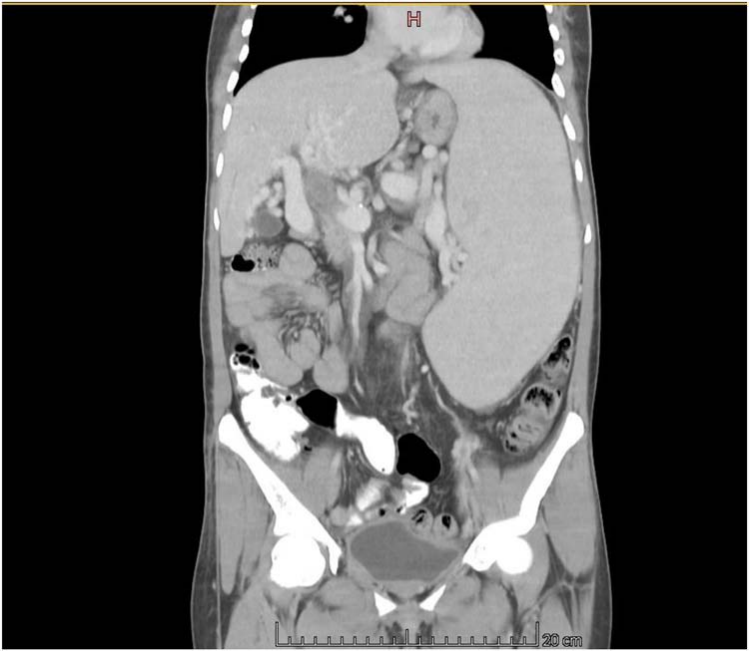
CT scan coronal view showing gross hepatosplenomegaly with large collaterals suggestive of portal venous hypertension with portal biliopathy. Chronic thrombus seen in SMV and portal confluence.
